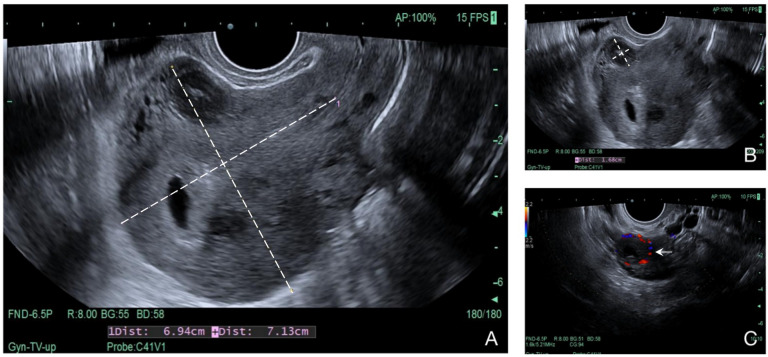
Ultrasound Results of case 2. (A) Uterine size showed by ultrasound. (6.94 cm × 7.13 cm). (B) The maximum size of leiomyomas (16 mm × 17 mm × 12 mm). (C) Extensive blood supply for the uterine leiomyomas.
